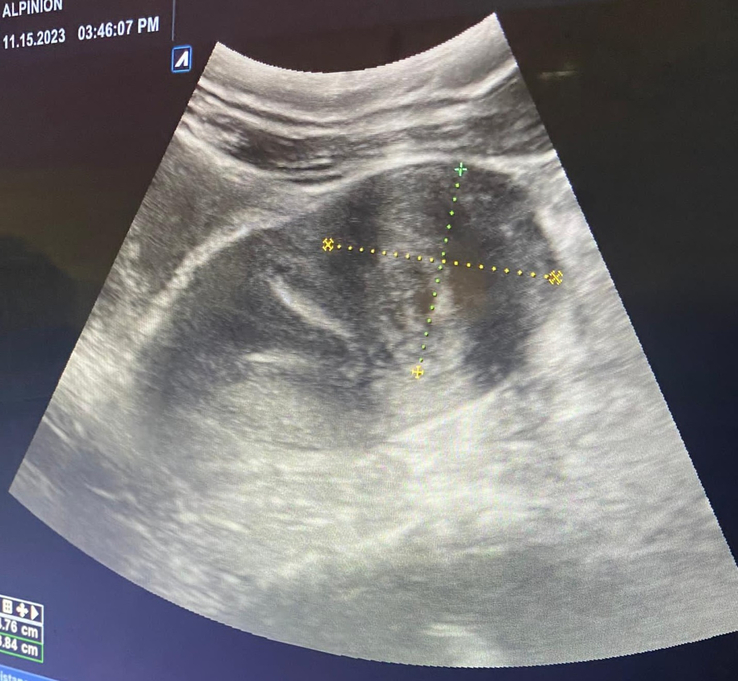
Pelvic ultrasound showing subserosal fibroid in the uterus.
